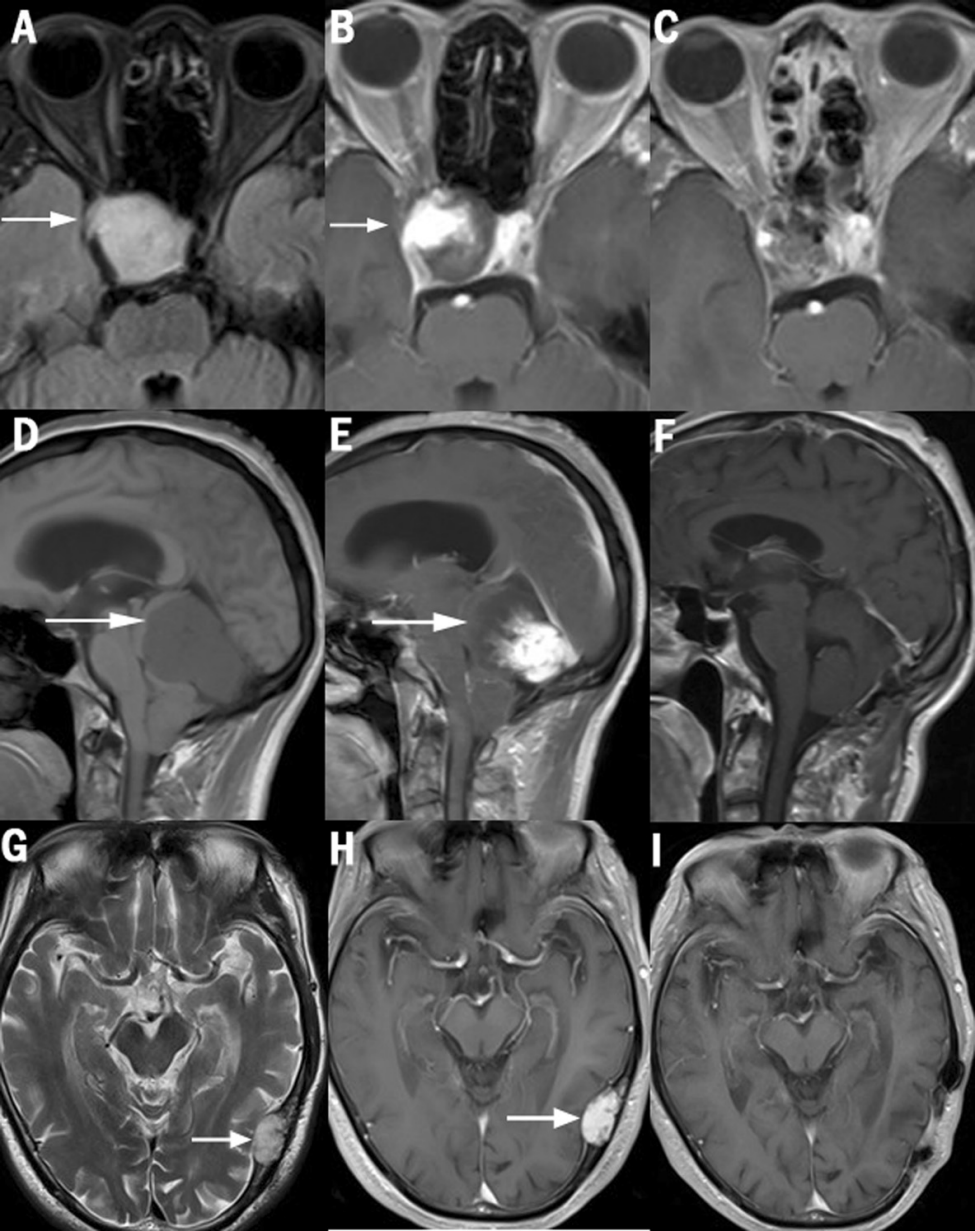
MRI scans for cases 1-3. Case 1: (A) preoperative MRI T2-w FLAIR, (B) T1-w MRI with contrast revealed a partially enhanced lesion in the sella, adjacent to the cavernous sinus, (C) postoperative MRI with contrast. Case 2: (D) preoperative MRI T1-w revealed a large subtentorial lesion, the cerebellum and brainstem were obviously compressed forward and downward, (E) MRI with contrast revealed the lesion was partially flame-like enhanced, (F) postoperative MRI with contrast one year later. Case 3: (G) preoperative T2-w MRI revealed a lesion in diploic space of the left temporal skull vault which had invaded inward, (H) MRI with contrast showed the tumor was enhanced, (I) postoperative MRI with contrast one year later. The arrows indicate the tumors. MRI (Magnetic resonance imaging), FLAIR (fluid-attenuated inversion recovery).
